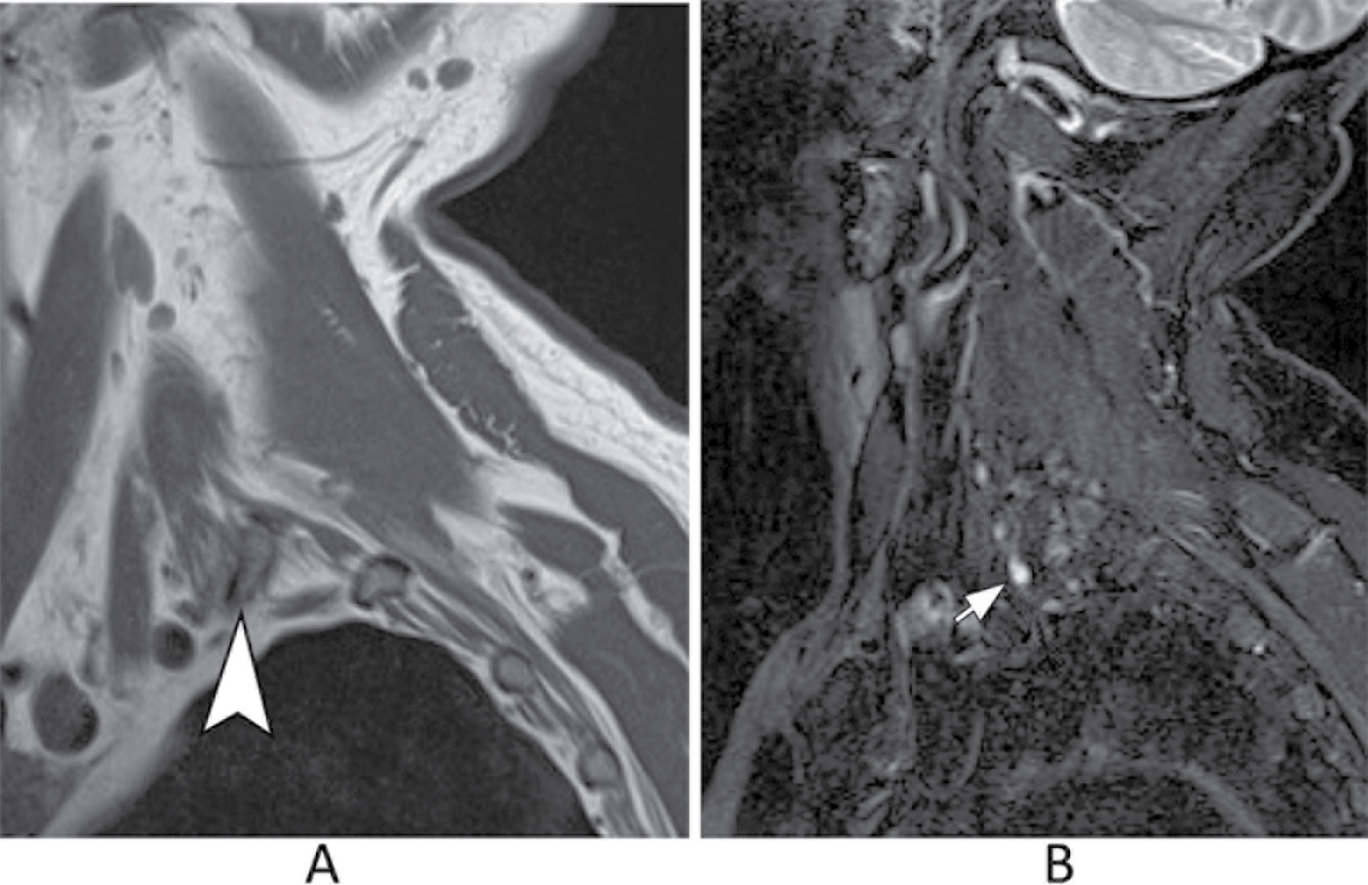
Thoracic outlet syndrome. Sagittal T1-weighted (A) and T2-weighted with fat suppression (B) images of the brachial plexus. There is a cervical rib (arrowhead) associated with thickening and increased signal of C8 nerve root, indicating neuropathy
